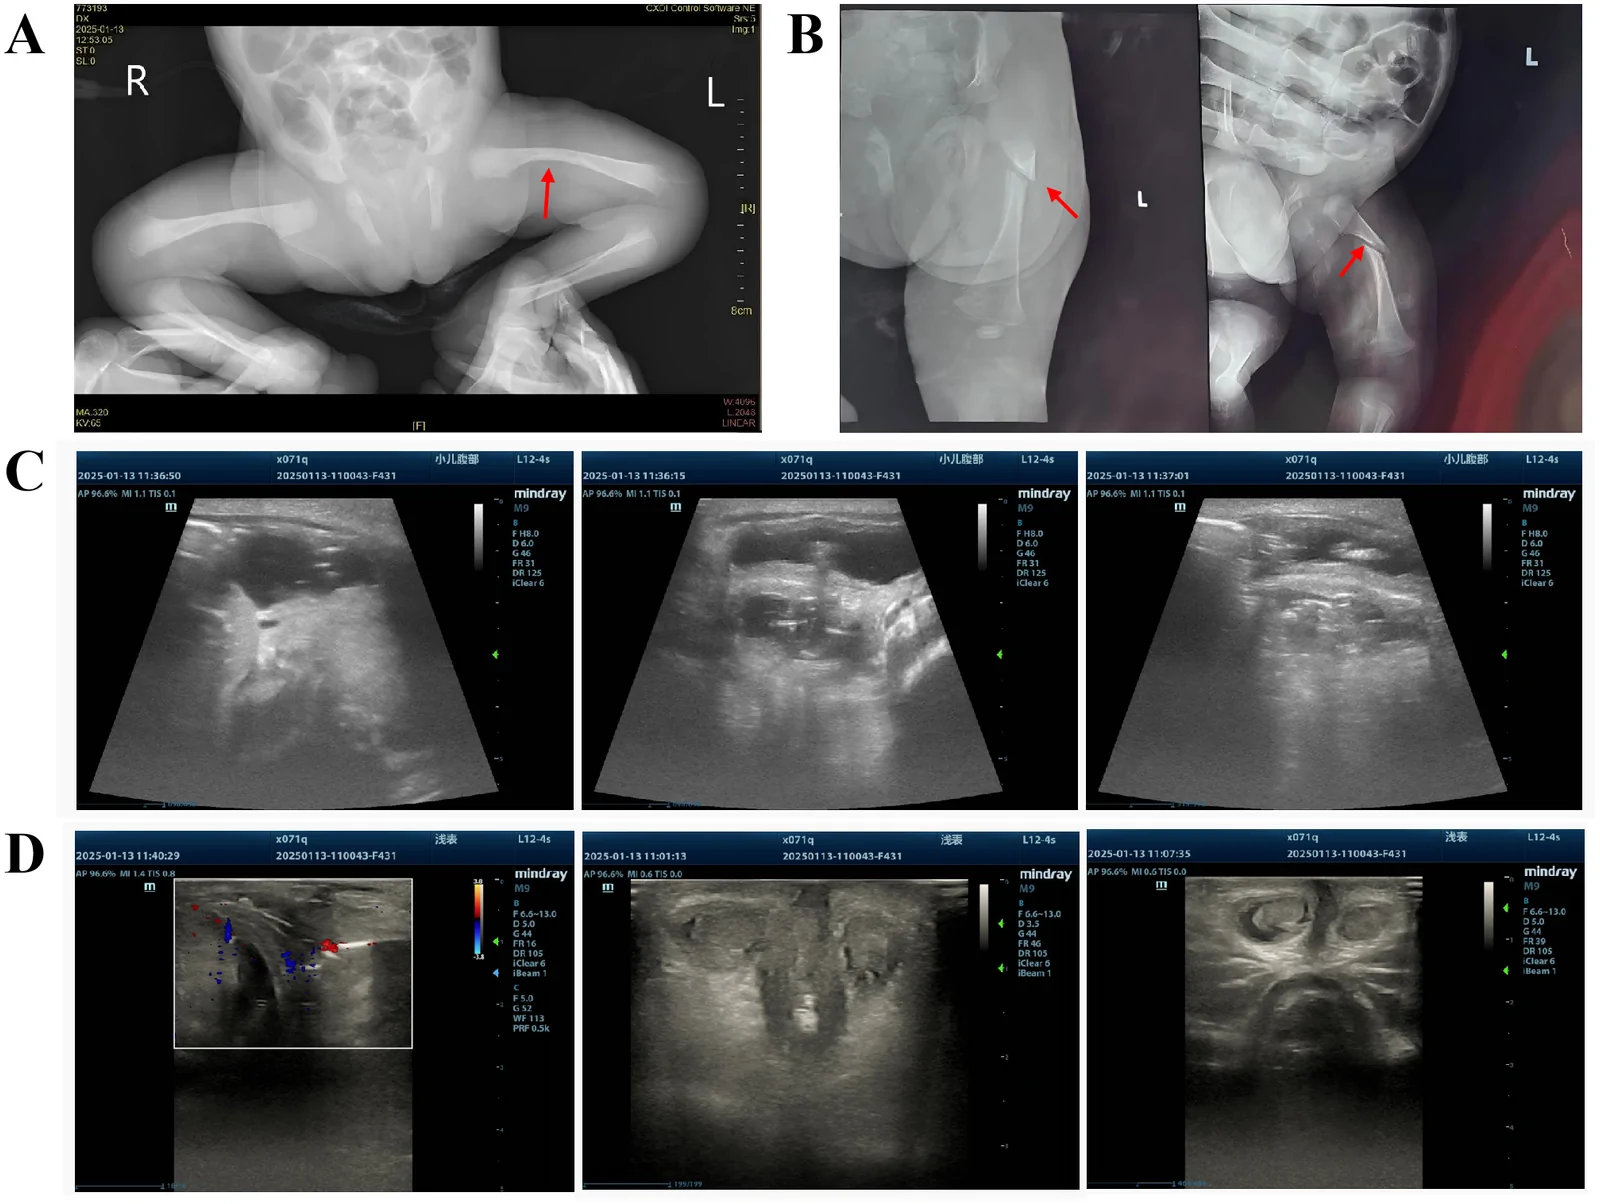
Imaging of the patient’s skeletal system, pelvis, and perineum. (A) Radiograph on day 1 after birth shows curvature of the left femur (arrow). (B) Radiograph on day 42 after birth shows a fracture of the left femur (arrow). Color Doppler ultrasound reveals no clear imaging of the uterus and ovaries (C), and a testis-like echo in the perineal region (D).
